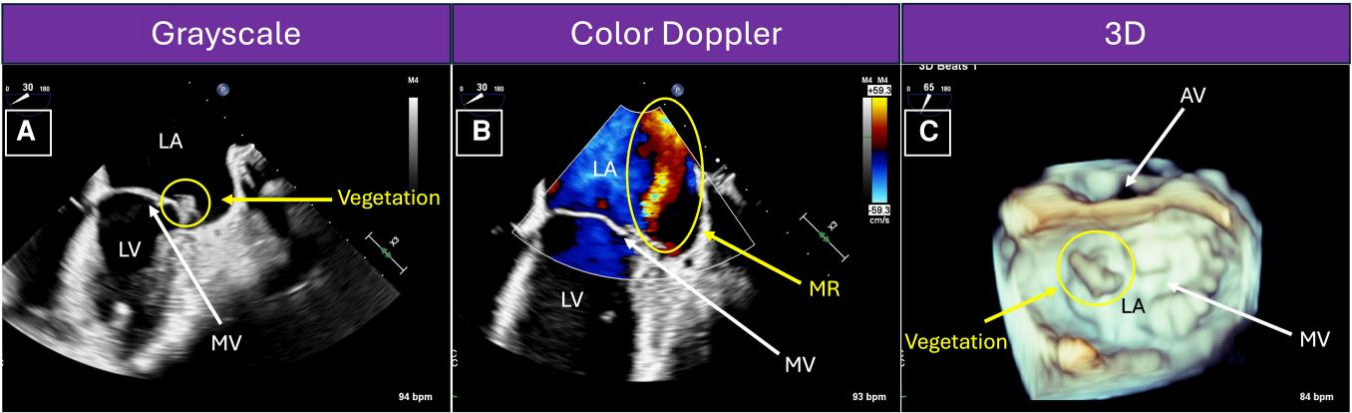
Case 2 (Mitral Valve Vegetation and Mitral Regurgitation): transoesophageal echocardiogram (TEE) with midesophageal two-chamber view at 30° in greyscale and colour Doppler and 3D TEE en face. TEE best visualizes the leaflets and segments of the mitral valve (*Panel A*), allowing for characterization of the vegetation and any related valvular abnormalities. The mitral regurgitation jet seen with colour Doppler (*Panel B*) appears at the exact location of the vegetation, as opposed to the coaptation point of the mitral valve leaflets. This is suggestive of a perforated leaflet. The 3D TEE reconstruction (*Panel C*) in the surgical view visualizes the relationship between vegetation and the other cardiac structures. This figure corresponds to Supplementary material online, *Video S6* and *S7*. AV, aortic valve; LA, left atrium; LV, left ventricle; MR, mitral regurgitation; MV, mitral valve.
